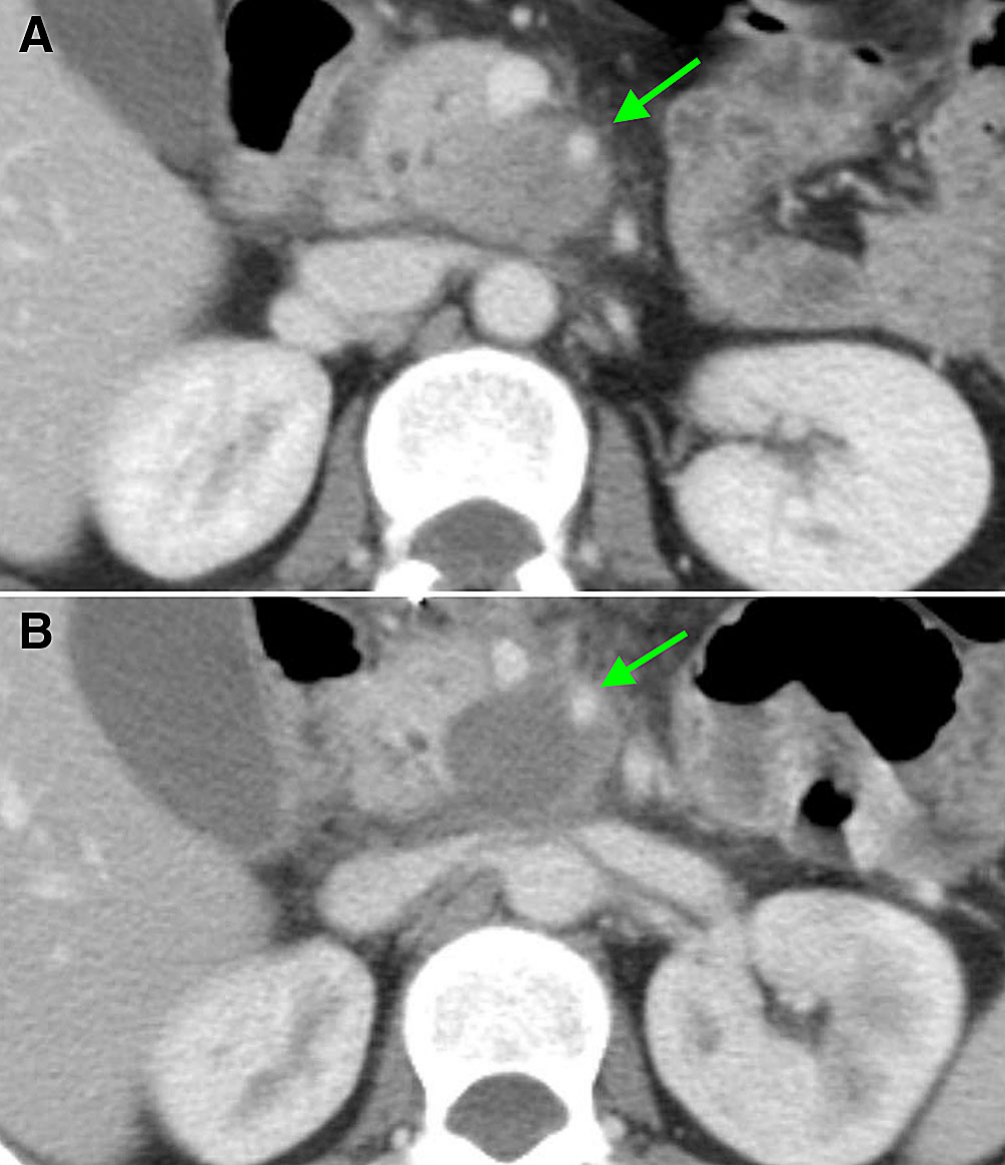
CT-images in the axial plane, of a 44-year-old woman with a ductal adenocarcinoma in the head of the pancreas. A On the pre-RFA CT-image, the superior mesenteric artery (SMA) was involved in the tumor, with a circumferential involvement of > 270, with normal patency and without anatomical changes. B On the CT-image 1-week post-RFA, the SMA was included in the ablation zone for 90°–180°, without the occurrence of anatomical changes
